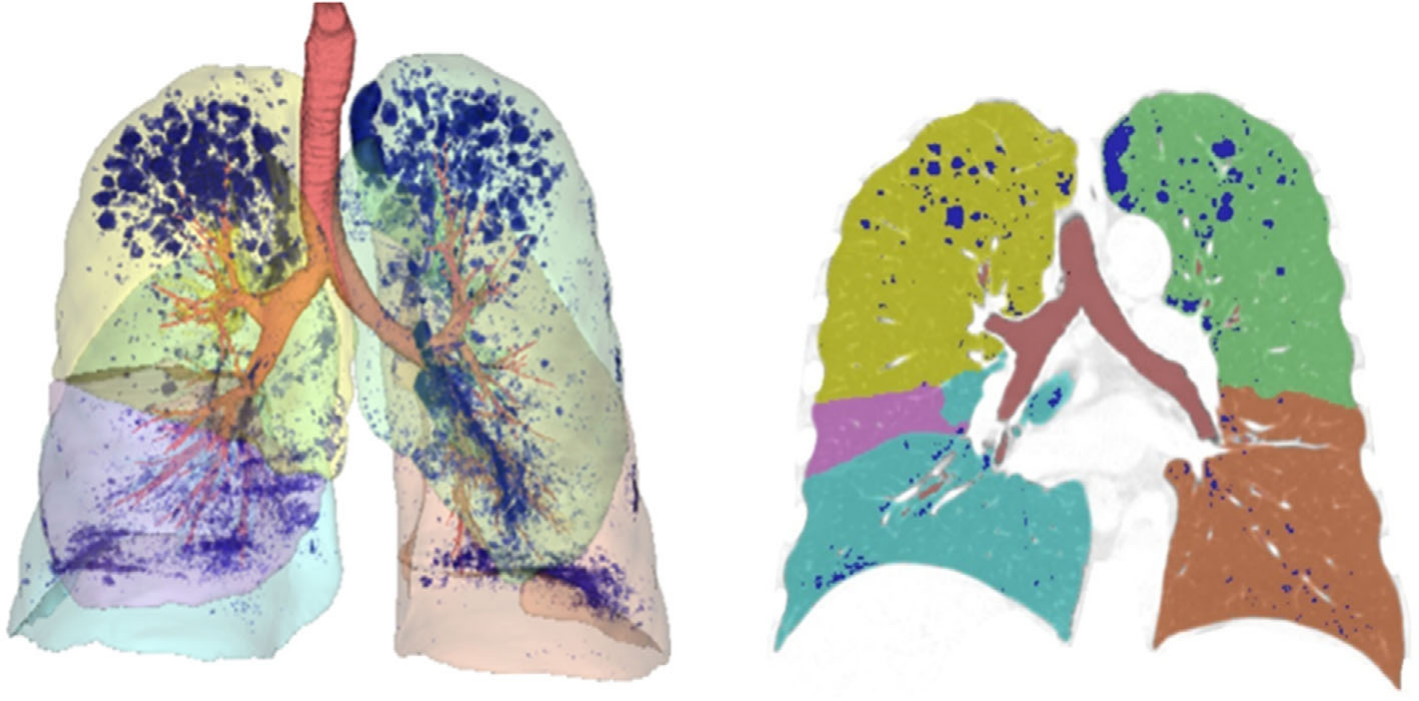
Example of low‐attenuation area in the lung on computed tomography (CT) examined using Ziostation2.
